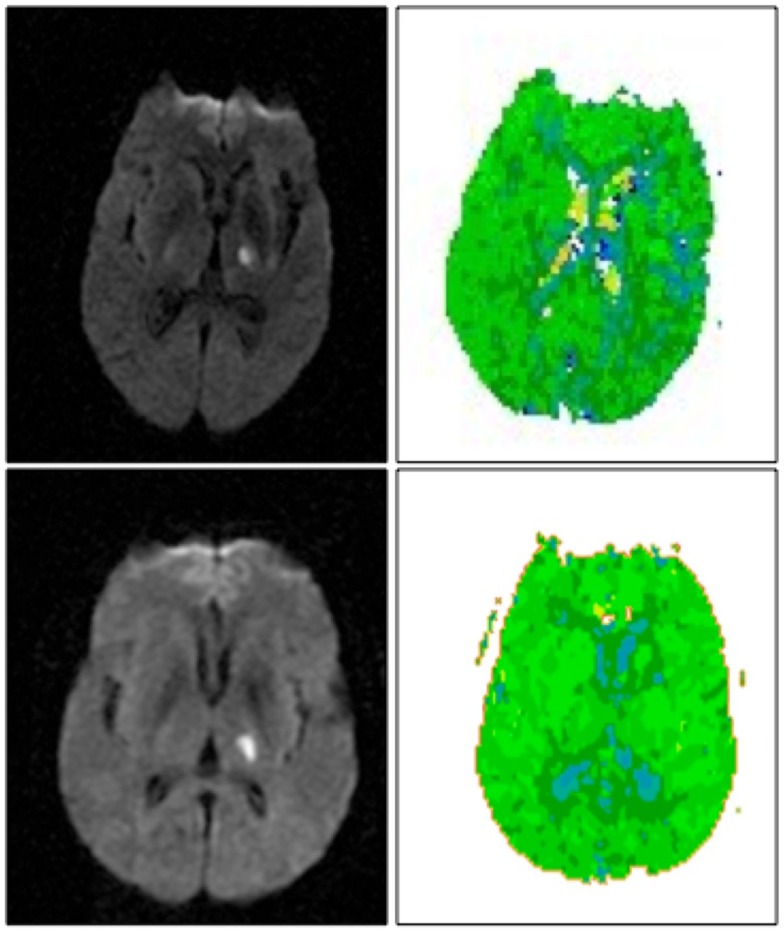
Left thalamic infarct with cortical hypoperfusion associated with cortical hypoperfusion (top) and without cortical hypoperfusion (bottom). DWI scans are shown on the left, PWI scans are shown on the right. Scans are in radiological convention (left hemisphere on right). Blue areas are hypoperfused.
